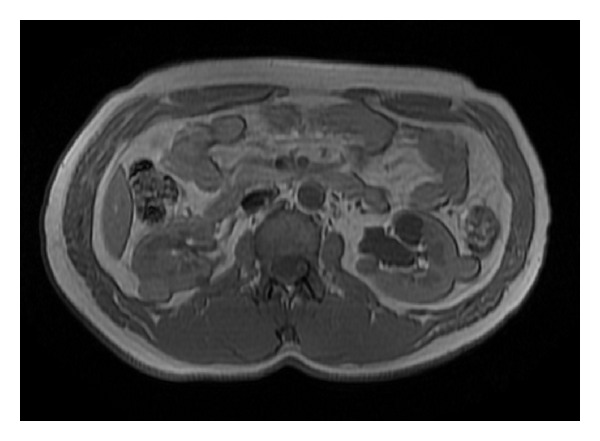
T1-weighted abdominal MRI in the axial plane demonstrating bilateral multiple renal masses of varying sizes and MR signal intensities.
